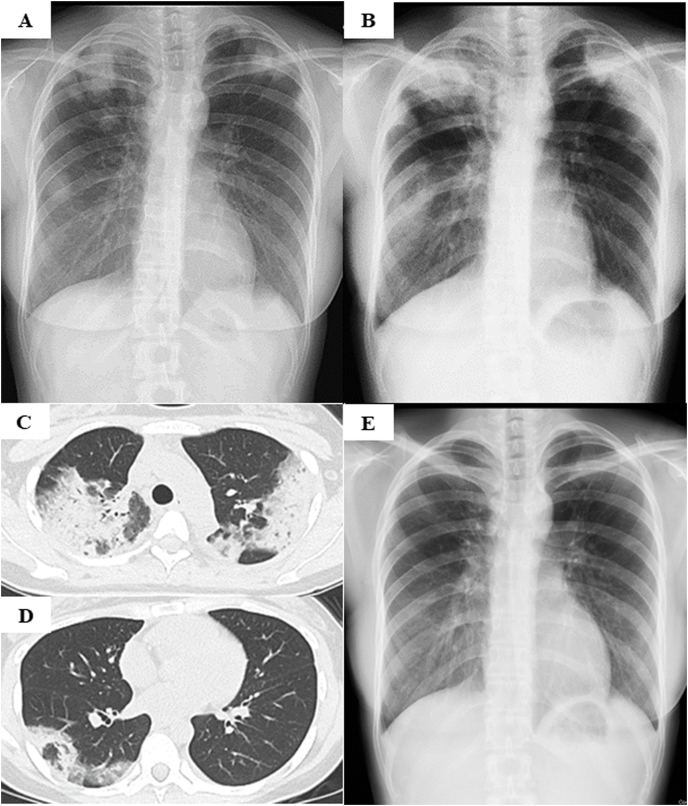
Chest radiograph showing the bilateral infiltrative shadows in upper lung fields (A). After 1 week of levofloxacin administration, the infiltrative shadows increased (B), and chest computed tomography show nonsegmental bilateral airspace consolidation in the peripheral region (C, D). After one month of TMP-SMX discontinuation, abnormal infiltrates almost disappeared (E). TMP-SMX; trimethoprim-sulfamethoxazole.
